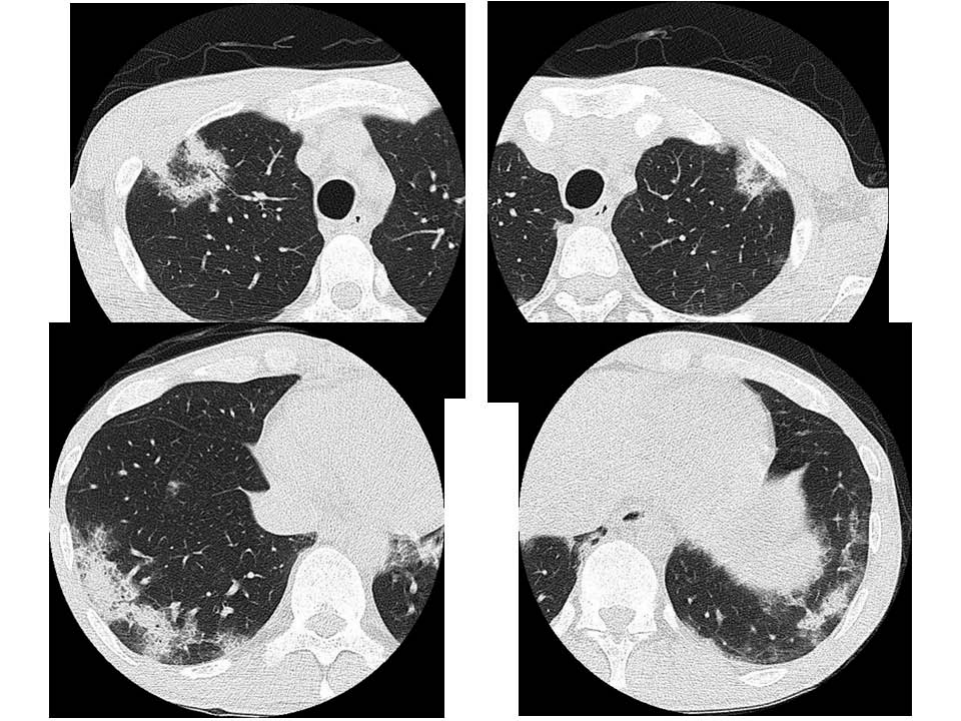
High-resolution computed tomography images of the lung before sulfamethoxazole trimethoprim treatment. The reversed halo signs and consolidations are shown.
